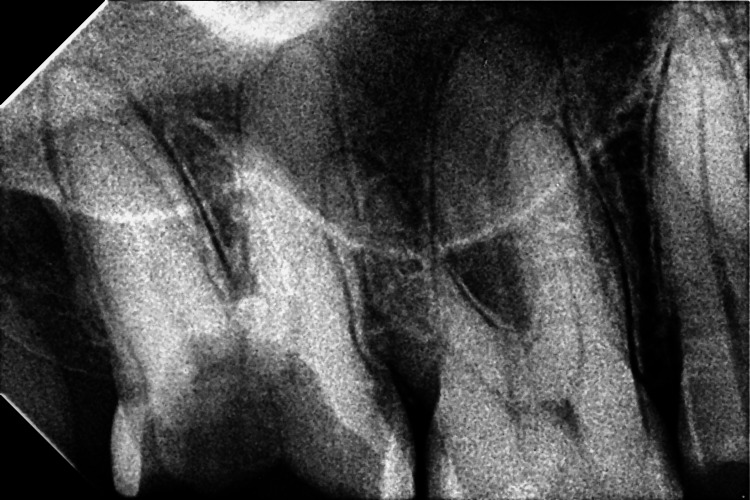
Shows preoperative radiograph with the upper right second molar
